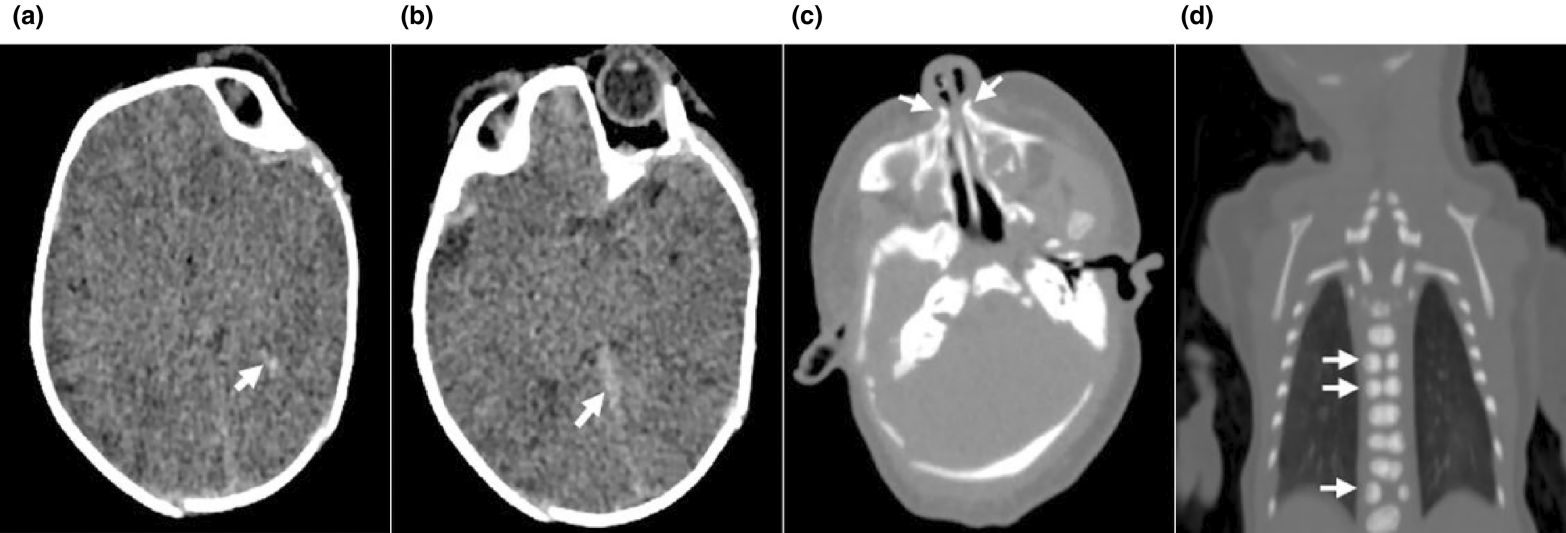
Computed tomography (CT) examination result of Proband 1. (a,b) Craniocerebral CT image of the patient's head, showing unclear demarcation between cerebral hemisphere gray and white matter and basal ganglia, linear high density seen in the posterior horn and posterior interhemispheric fissure of the left ventricle (arrow). (c) CT of the nasopharynx showing pyriform foramen stenosis (arrows). (d) CT of the thoracic spine showing increased bone density in the ribs, bilateral scapulae, and thoracic spine, and many vertebral bodies showed “sphenoid” deformity (arrows).
